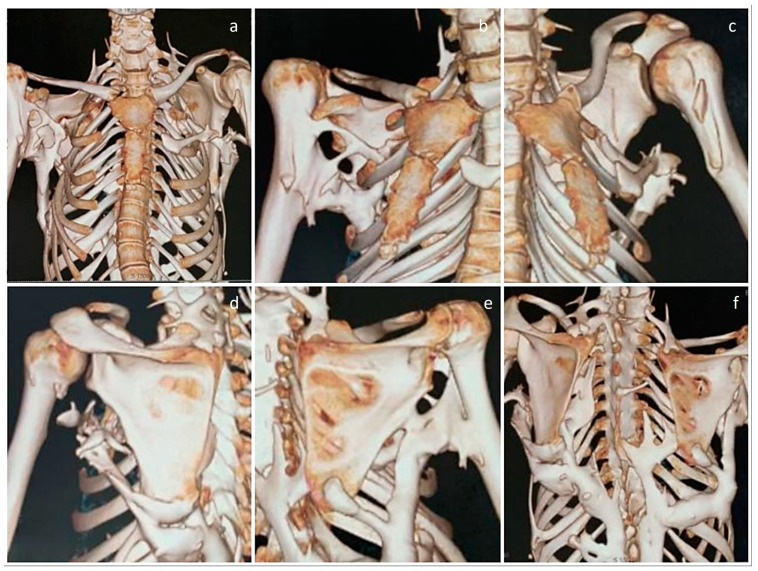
Close-up 3D CT images of the rib cage, scapulae, and shoulder joints. (a) While the front of the chest wall appears to be relatively spared, there is an isolated mass of heterotopic bone on the left anterior chest wall adjacent to the sternum. (b) Oblique view of the chest wall, showing fusion of the right humerus to the heterotopic bone mass in the posterior aspect. (c,d) Oblique view, showing that the left shoulder joint and humerus are free of restricting ossifications. (d,e) Posterior views, showing that both scapulae are fused with the heterotopic bone extending from the lumbar paraspinal region. (e) Fusion of the right humerus to the heterotopic bone mass can be noted. (f) Posterior view of the chest wall, clearly showing fusion of the posterior elements of the spine.
